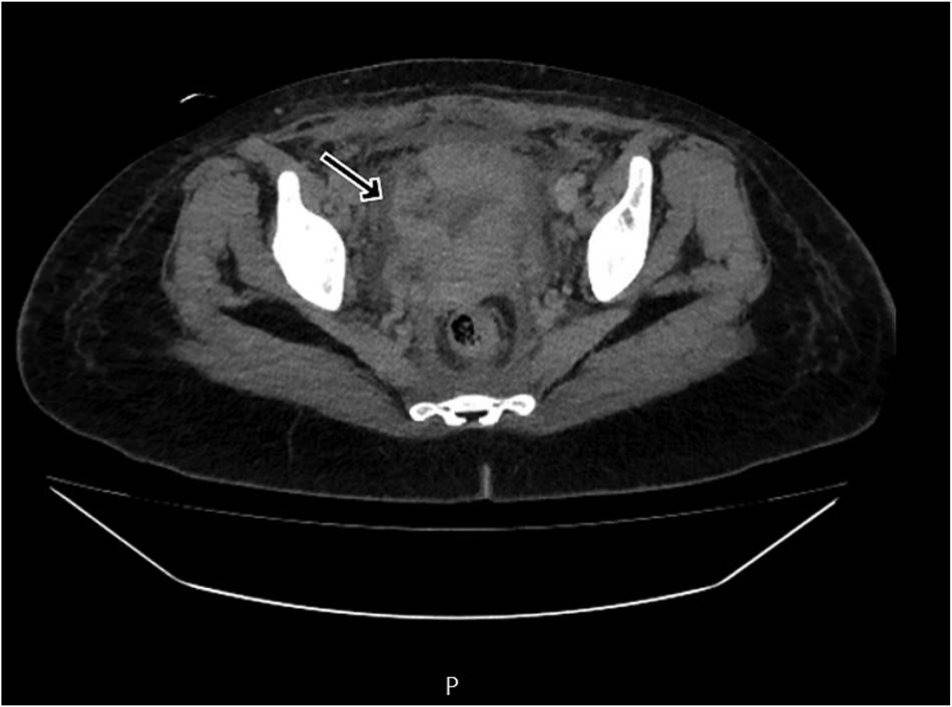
CT scan revealing a ruptured uterine scar with omental herniation.
